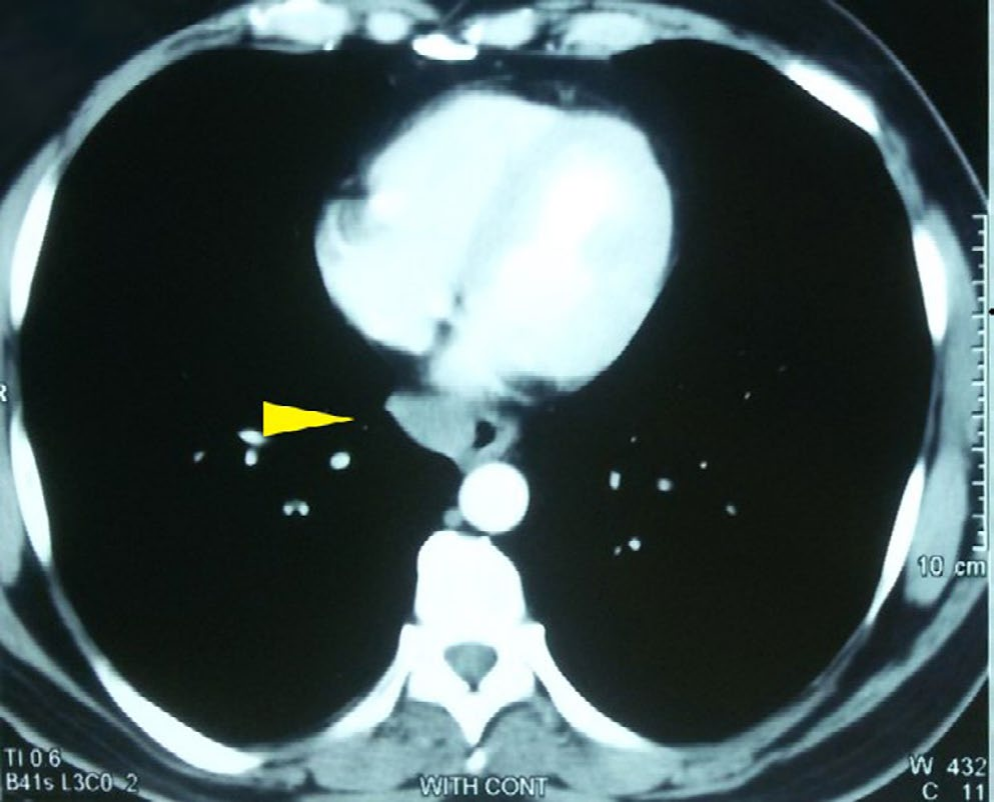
Contrast‐enhanced computed tomography of the chest showing a well‐defined homogeneous mass (yellow arrow head) on the right side of the distal esophagus
